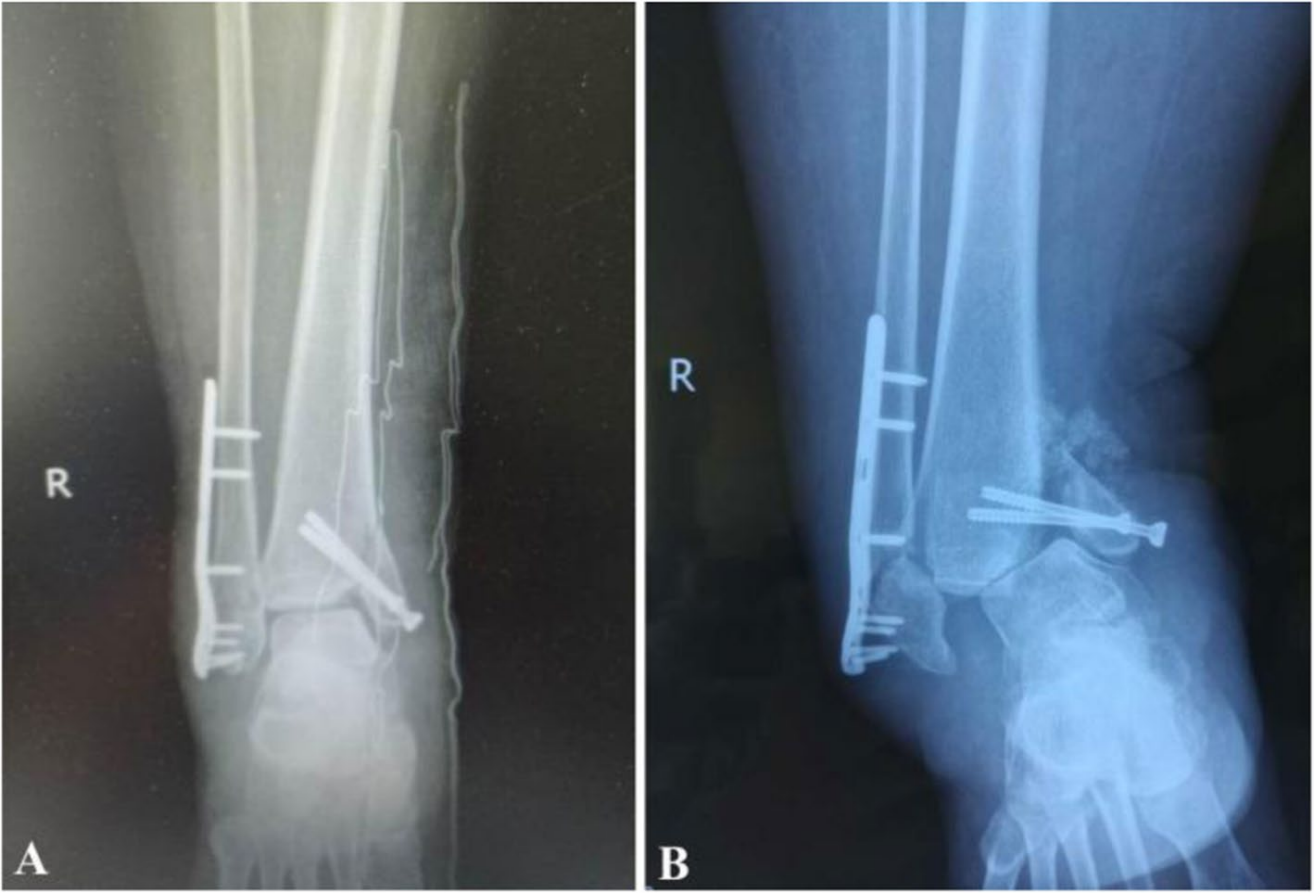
A typical case of internal fixation failure. A. Postoperative radiographs showing that the medial malleolus was only fixed with hollow screws, with no anti-skid plates or screws used for fixation. The collapse of the medial malleolus was not adequately treated with bone grafting. B. Two months after the operation, the internal fixation was found to have failed after a sprain while walking
